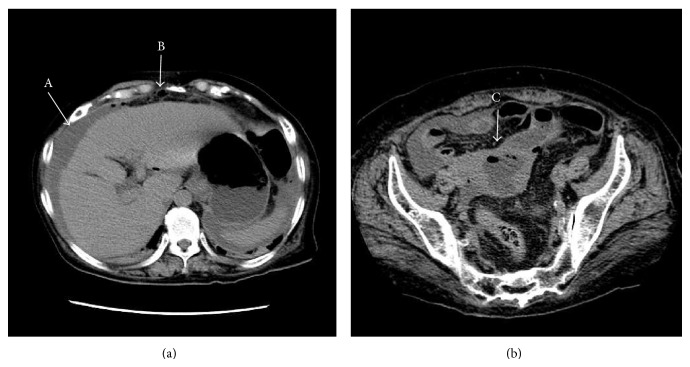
Transverse views of computed tomography showing the presence of fluid (A) and free air (B) in the upper abdomen (a) and a fluid filled uterus and intrauterine free air (C) (b).
